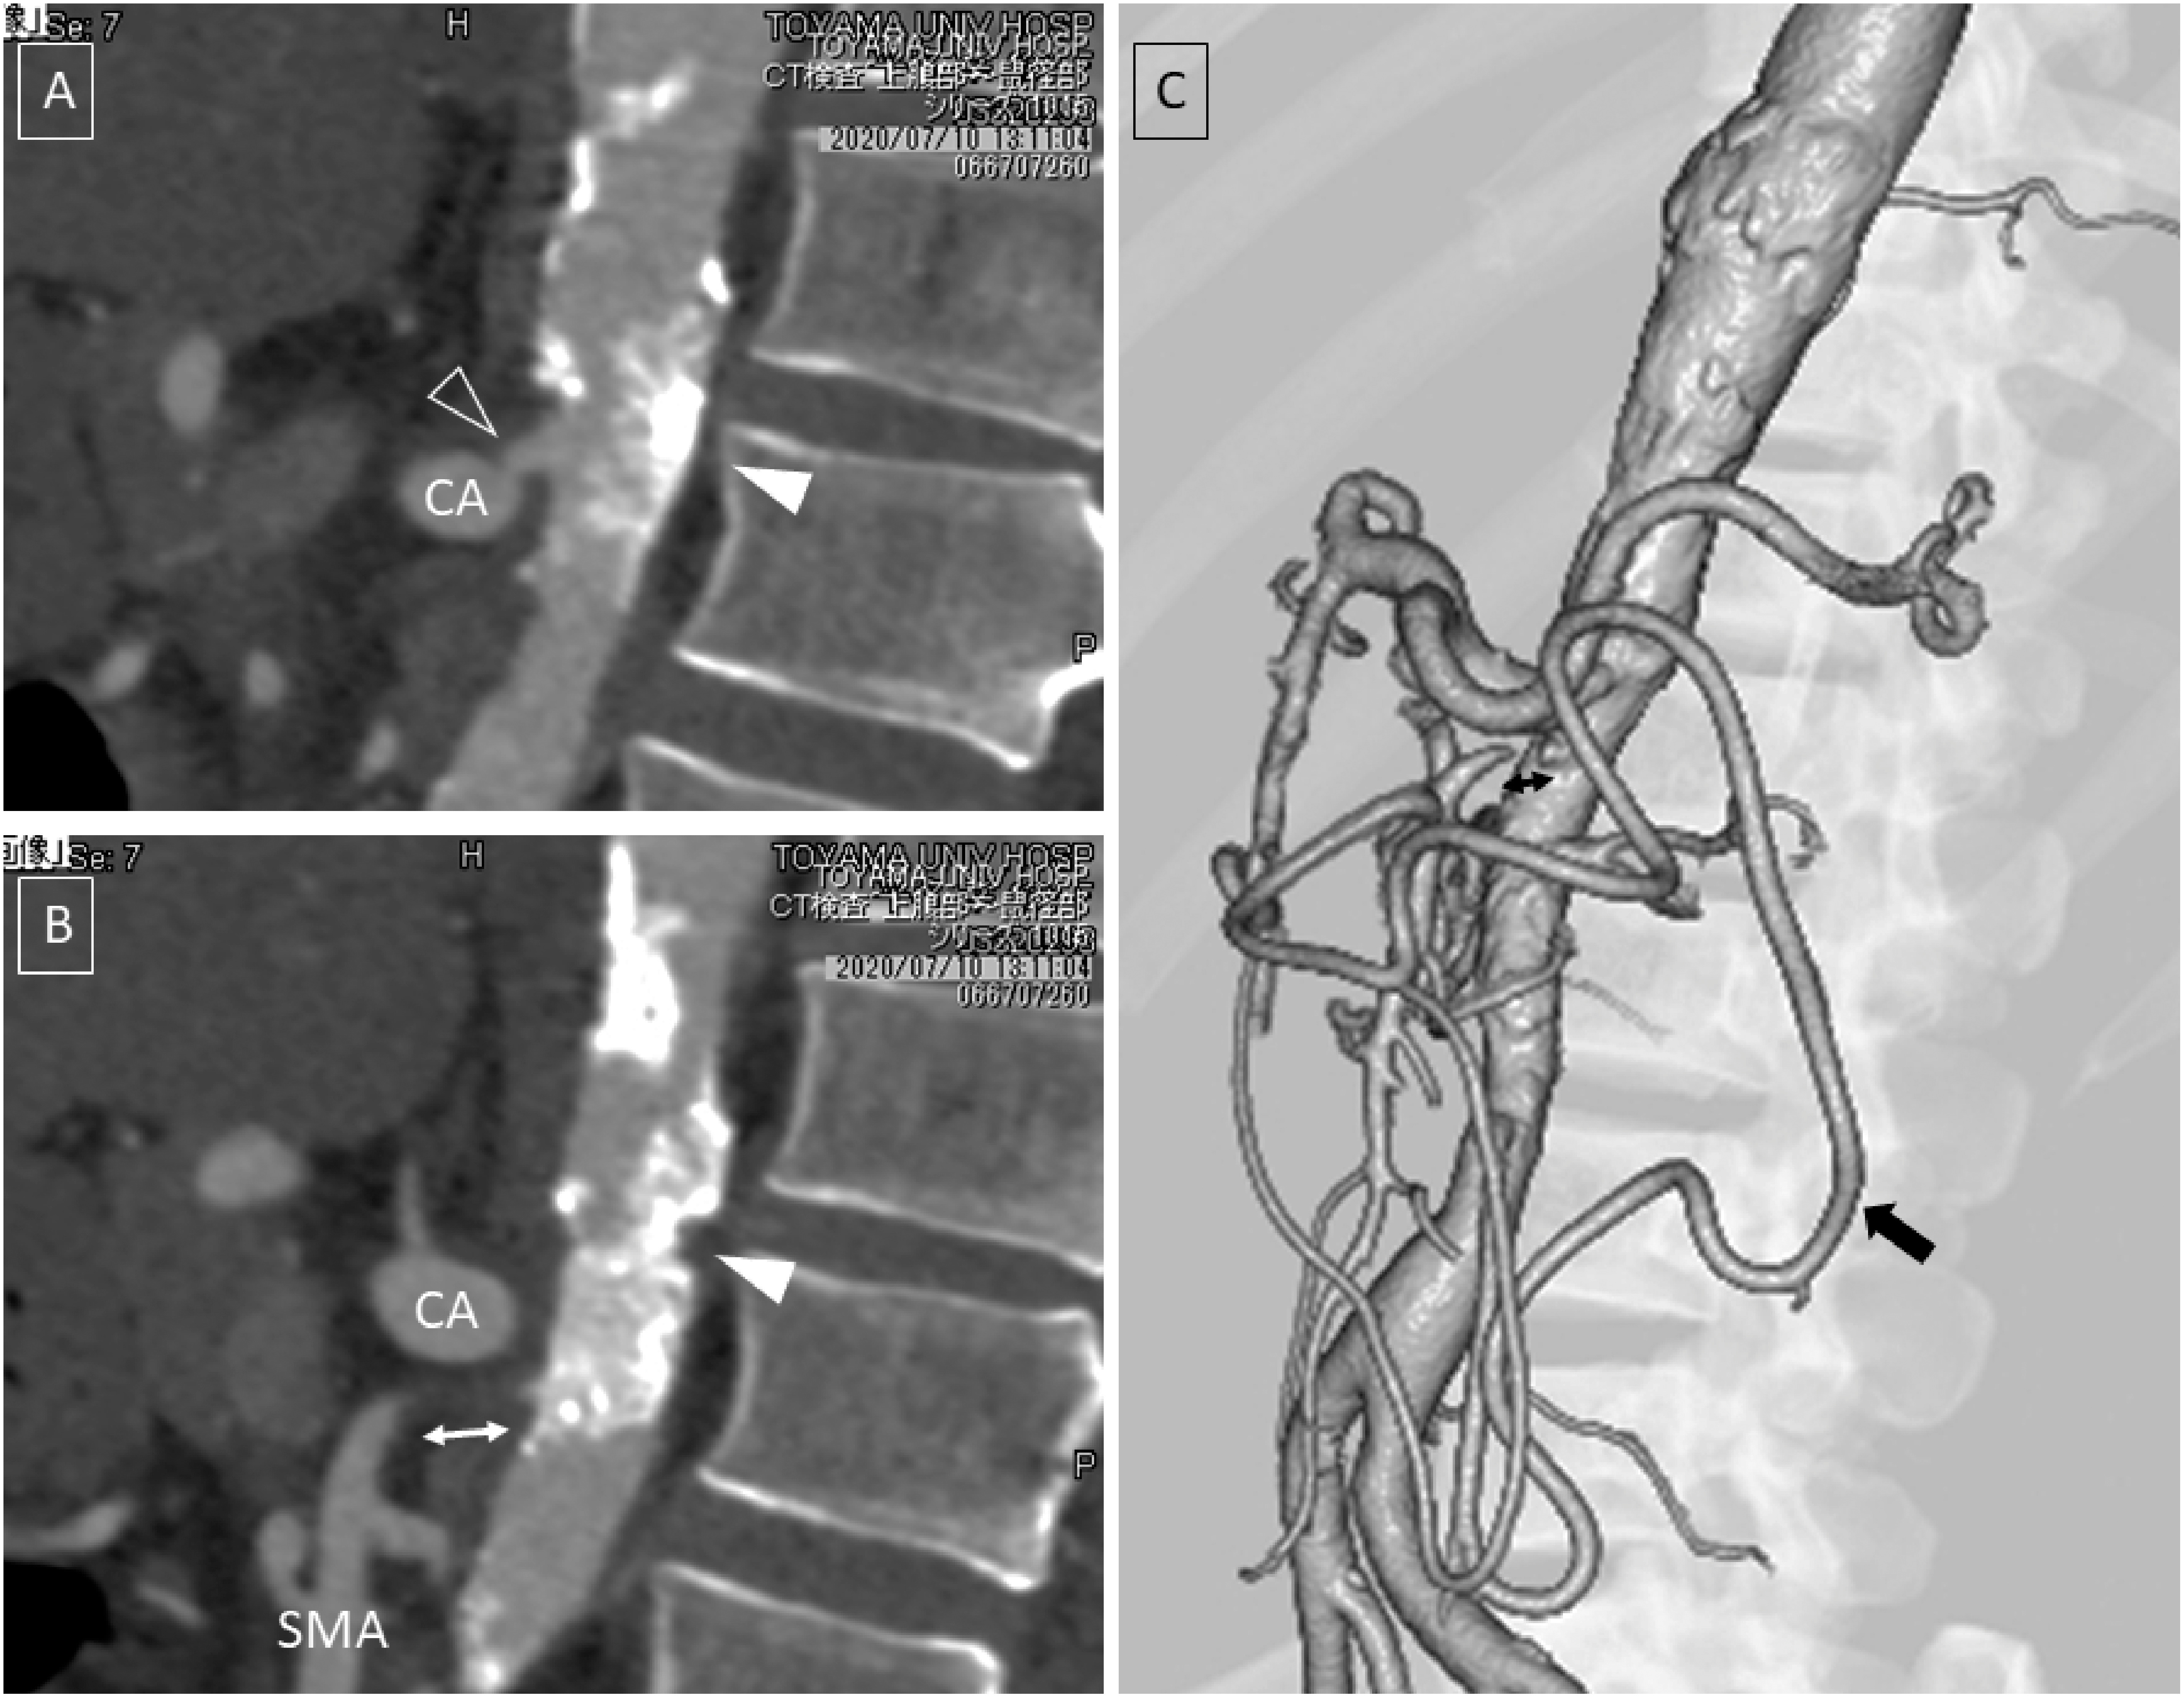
Fig. 2 Preoperative enhanced computed tomography (A and B, a sagittal view; C, a volume-rendered image). (A) Calcified stenosis in the abdominal aorta (filled arrowhead) and stenosis of origin in the celiac artery (unfilled arrowhead). (B) Calcified stenosis in the abdominal aorta (filled arrowhead) and occlusion in the superior mesenteric artery (SMA) (double-headed arrow). (C) Collateral blood vessels from the developed inferior mesenteric artery to the SMA can be seen (arrow), and the SMA is occluded (double-headed arrow).
